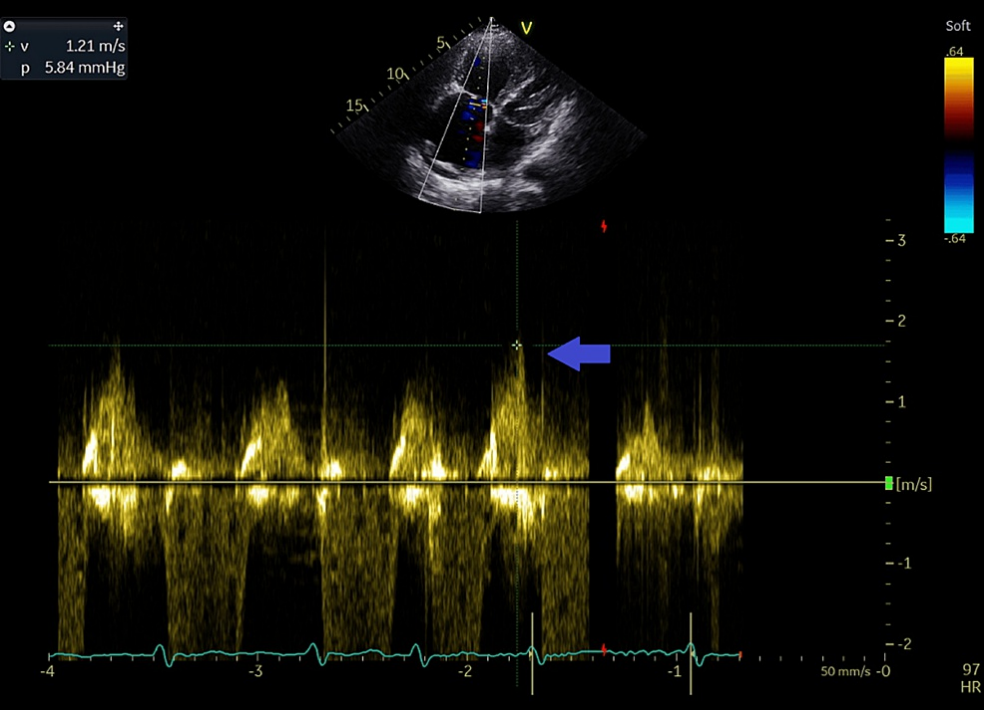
Tricuspid E velocity by continuous wave Doppler recorded at 1.2 m/second from apical four-chamber view. Blue arrow shows the E velocity spectral display
